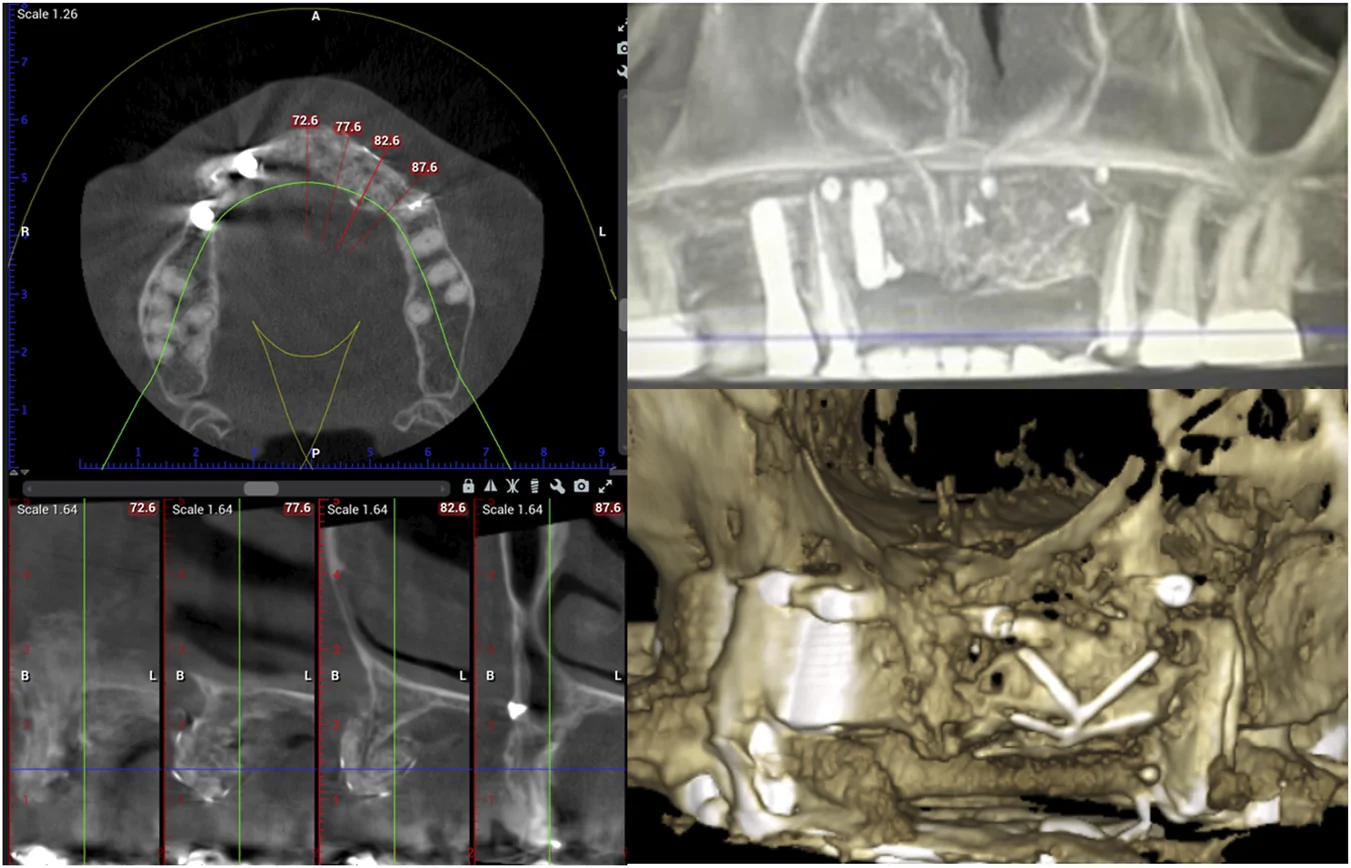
Case 1: 6 months post-grafting. Images show increased bone volume at grafted sites resulting in increased ridge height and width. Top left, CBCT axial view. Bottom left, coronal view. Top right, panoramic radiograph. Bottom right, 3D reconstruction.
